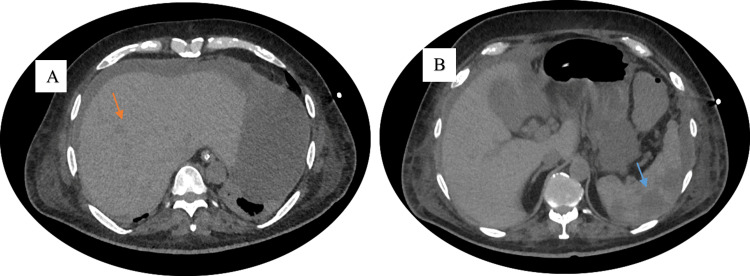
Contrast-Enhanced CT Abdomen on Hospital Day 32 Showing Hepatic and Splenic Hypodense Lesions Contrast-enhanced computed tomography (CT) of the abdomen performed on hospital day 32 reveals disseminated visceral involvement in a neutropenic patient with *Saprochaete capitata* fungemia. (A) Multiple hypodense lesions in the liver (orange arrow), suggestive of fungal microabscesses or infarcts. (B) Multiple hypodense lesions in the spleen (blue arrow), also indicative of fungal microabscesses or infarcts.
